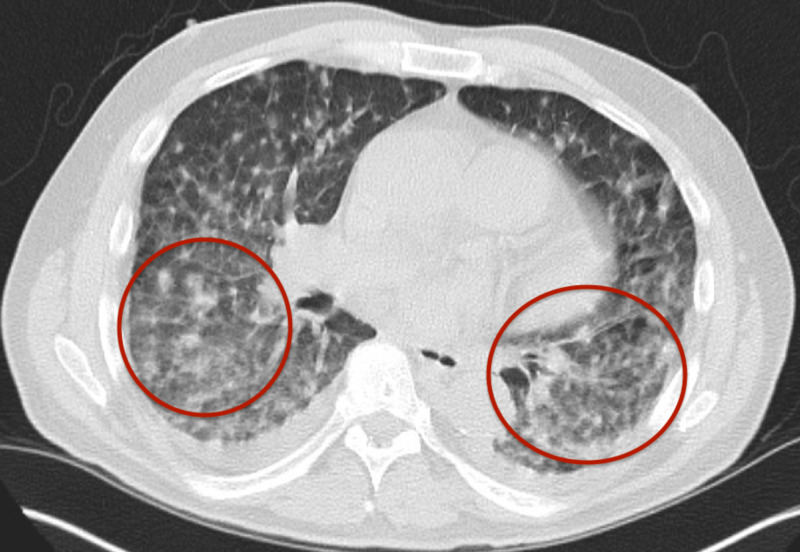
CT of the chest showing multiple bilateral septic emboli
